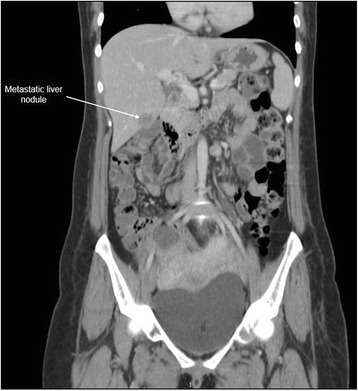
Initial coronal CT showing normal appearance of bowel and liver metastasis
